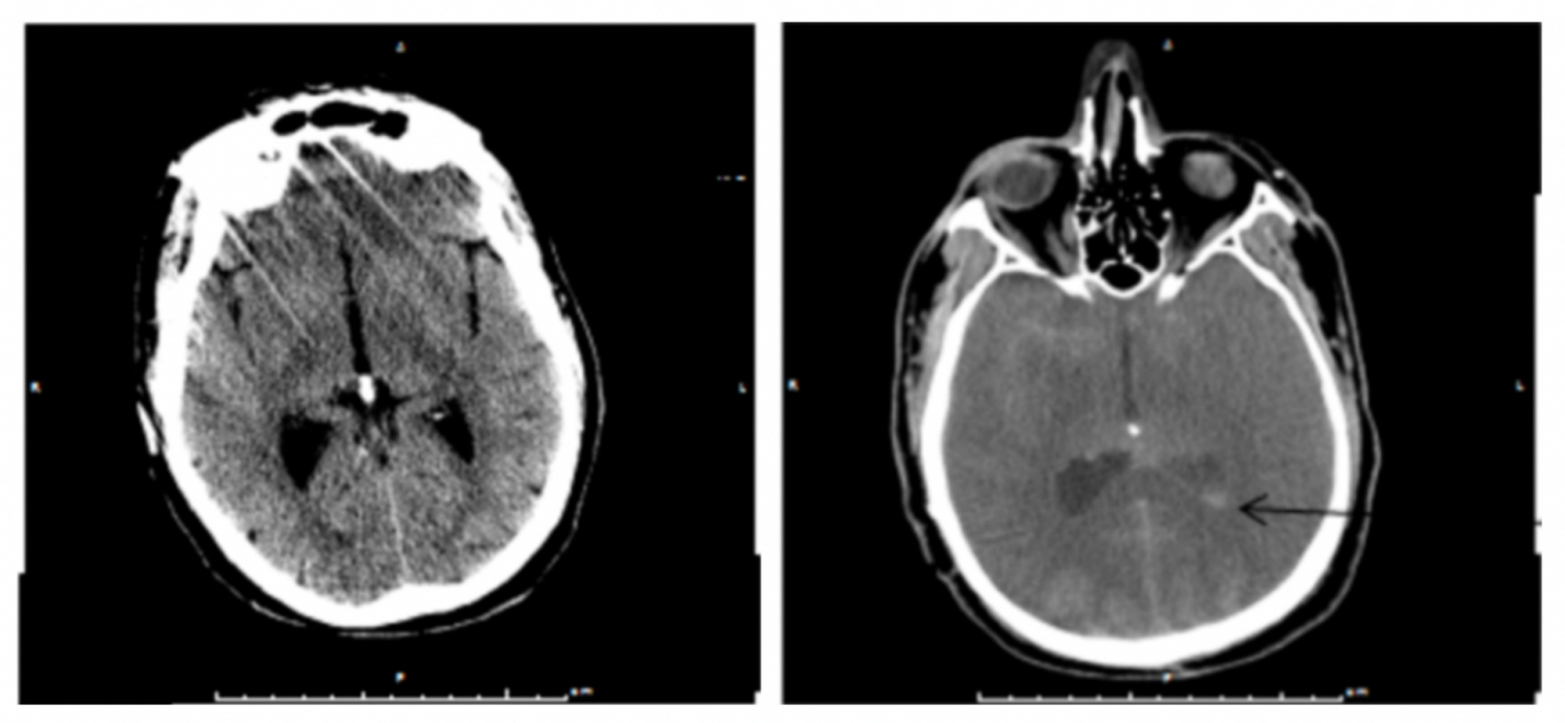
Initial head CT on presentation showing no subarachnoid hyperdensity (left); six-hour interval head CT showing intraventricular hyperdensity (arrow, right) CT: computed tomography
